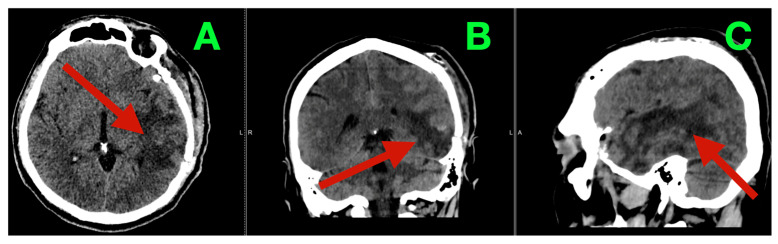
Control CT (postoperative day seven). (A) Axial non-contrast CT showing stable left temporo-basal postoperative cavity morphology (arrow), with resolving pneumocephalus and no delayed intraparenchymal hemorrhage. (B) Coronal reconstruction demonstrating maintained decompression of the temporal compartment (arrow), stable midline/ventricular anatomy, and no new extra-axial collection. (C) Sagittal reconstruction confirming durable skull base–parallel cavity geometry (arrow) without progressive edema, hemorrhagic conversion, or hydrocephalus.
